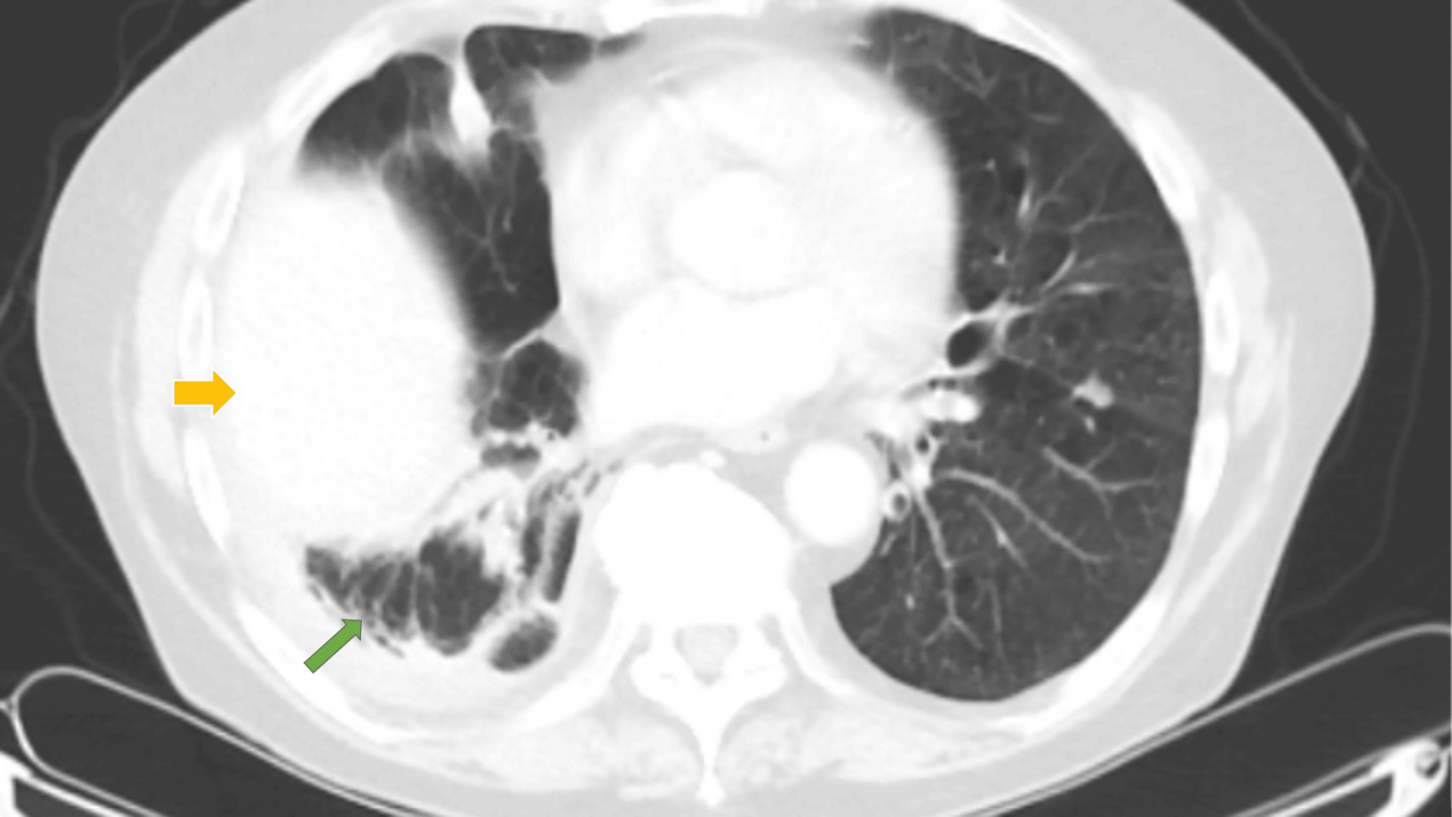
Follow-up CT of the thorax with contrast (25th June, 2025) showed interval improvement in right-sided empyema (yellow arrow), with a small residual loculated pleural collection and associated pleural thickening (green arrow).
